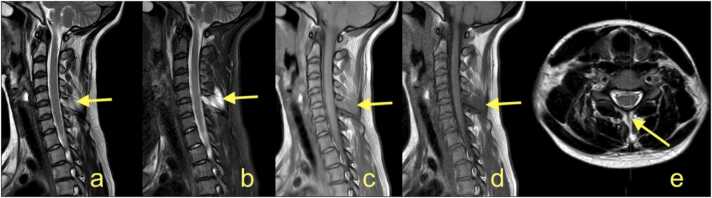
The cervical spine magnetic resonance imaging protocol used at the authors' institution is included in the examination protocol of the brachial plexus. (a) T2-weighted turbo spin-echo (TSE) sequence, (b) short tau inversion-recovery (STIR) sequence, (c) proton density-weighted TSE sequence, (d) T1-weighted TSE sequence, and (e) T2-weighted TSE sequence. A 24-year-old patient was involved in a car accident. There was complete paralysis of the C4-C8 segments, partially of the T1 segment in the motor fibers. MRI showed a rupture of the interspinous ligament (arrow) and the ligament flavum. Continued in Fig. 2.
